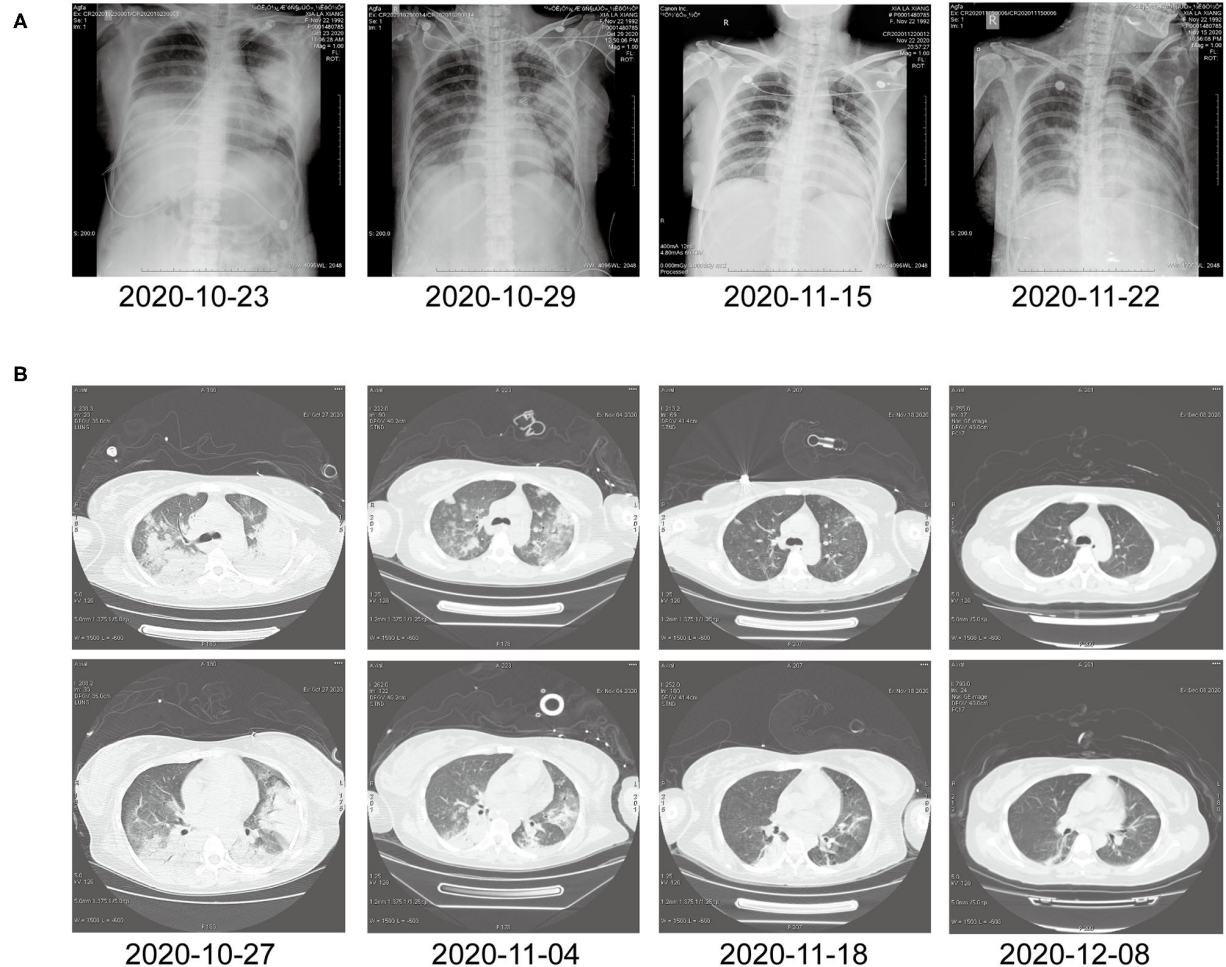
(A) Chest X-ray before and after treatment. (B) Chest computed tomography at different time points after treatment.
